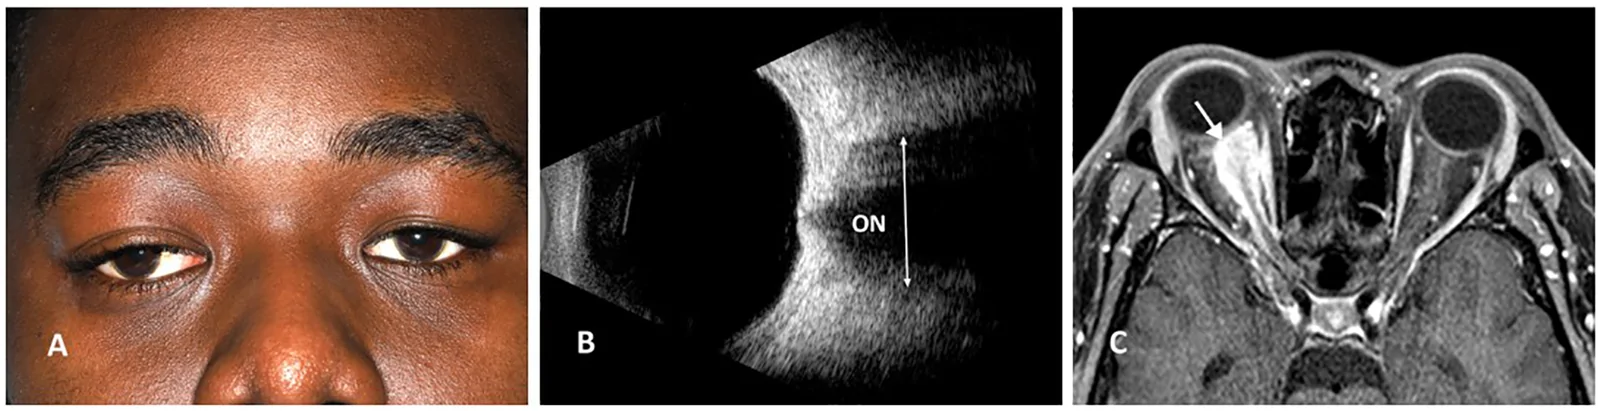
Optic Nerve Meningioma. A 24-year-old male presented with severe vision loss in the right eye for six months. (A) External photography shows proptosis and inferior displacement of the right eye. (B) Transverse B-scan shows a tubular, medium reflective enlargement of the optic nerve due to the enlargement of the meningeal sheath, with normal central neural component (double arrow). (C) Axial T1-weighted MRI shows an enhanced meningeal tumor surrounding the non-enhanced optic nerve matter centrally, resulting in the so-called tram-track sign (arrow). *ON*, optic nerve.
